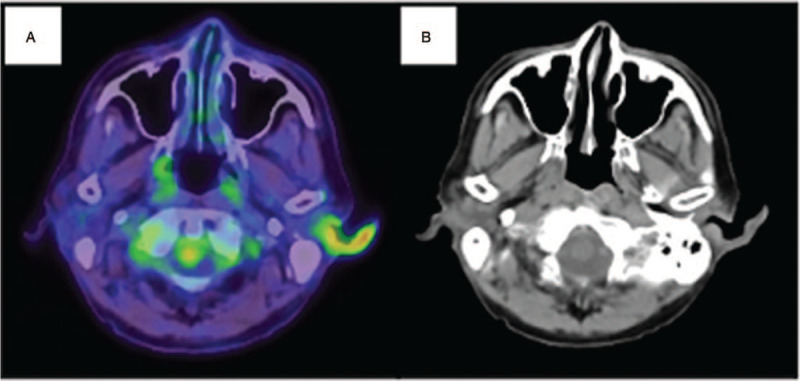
Finding of 18F-FDG positron emission tomography-computed tomography (PET-CT). (A) 18F-FDG PET-CT revealed strong accumulation in both auricles. (B) Metabolic activity lesion was corresponded to mild skin thickening on the CT.
